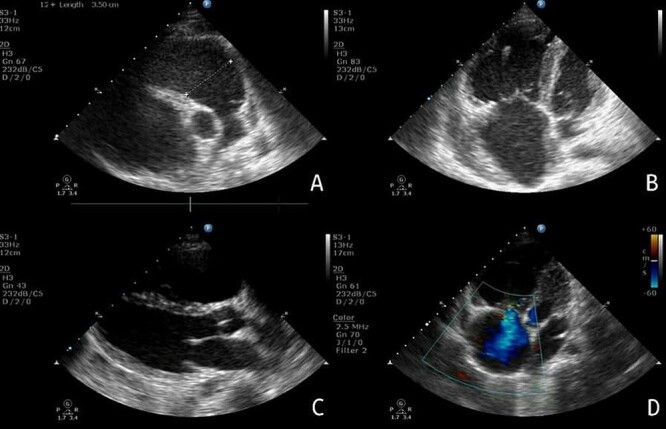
Echocardiography: (A) PSAX view, showing the extent of RV enlargement (PSAX RVOT = 35 mm). (B) Apical view of the four chambers, showing enlargement of the right-side chambers. (C) PLAX view. (D) Apical view of the four chambers, showing tricuspid valve insufficiency.
